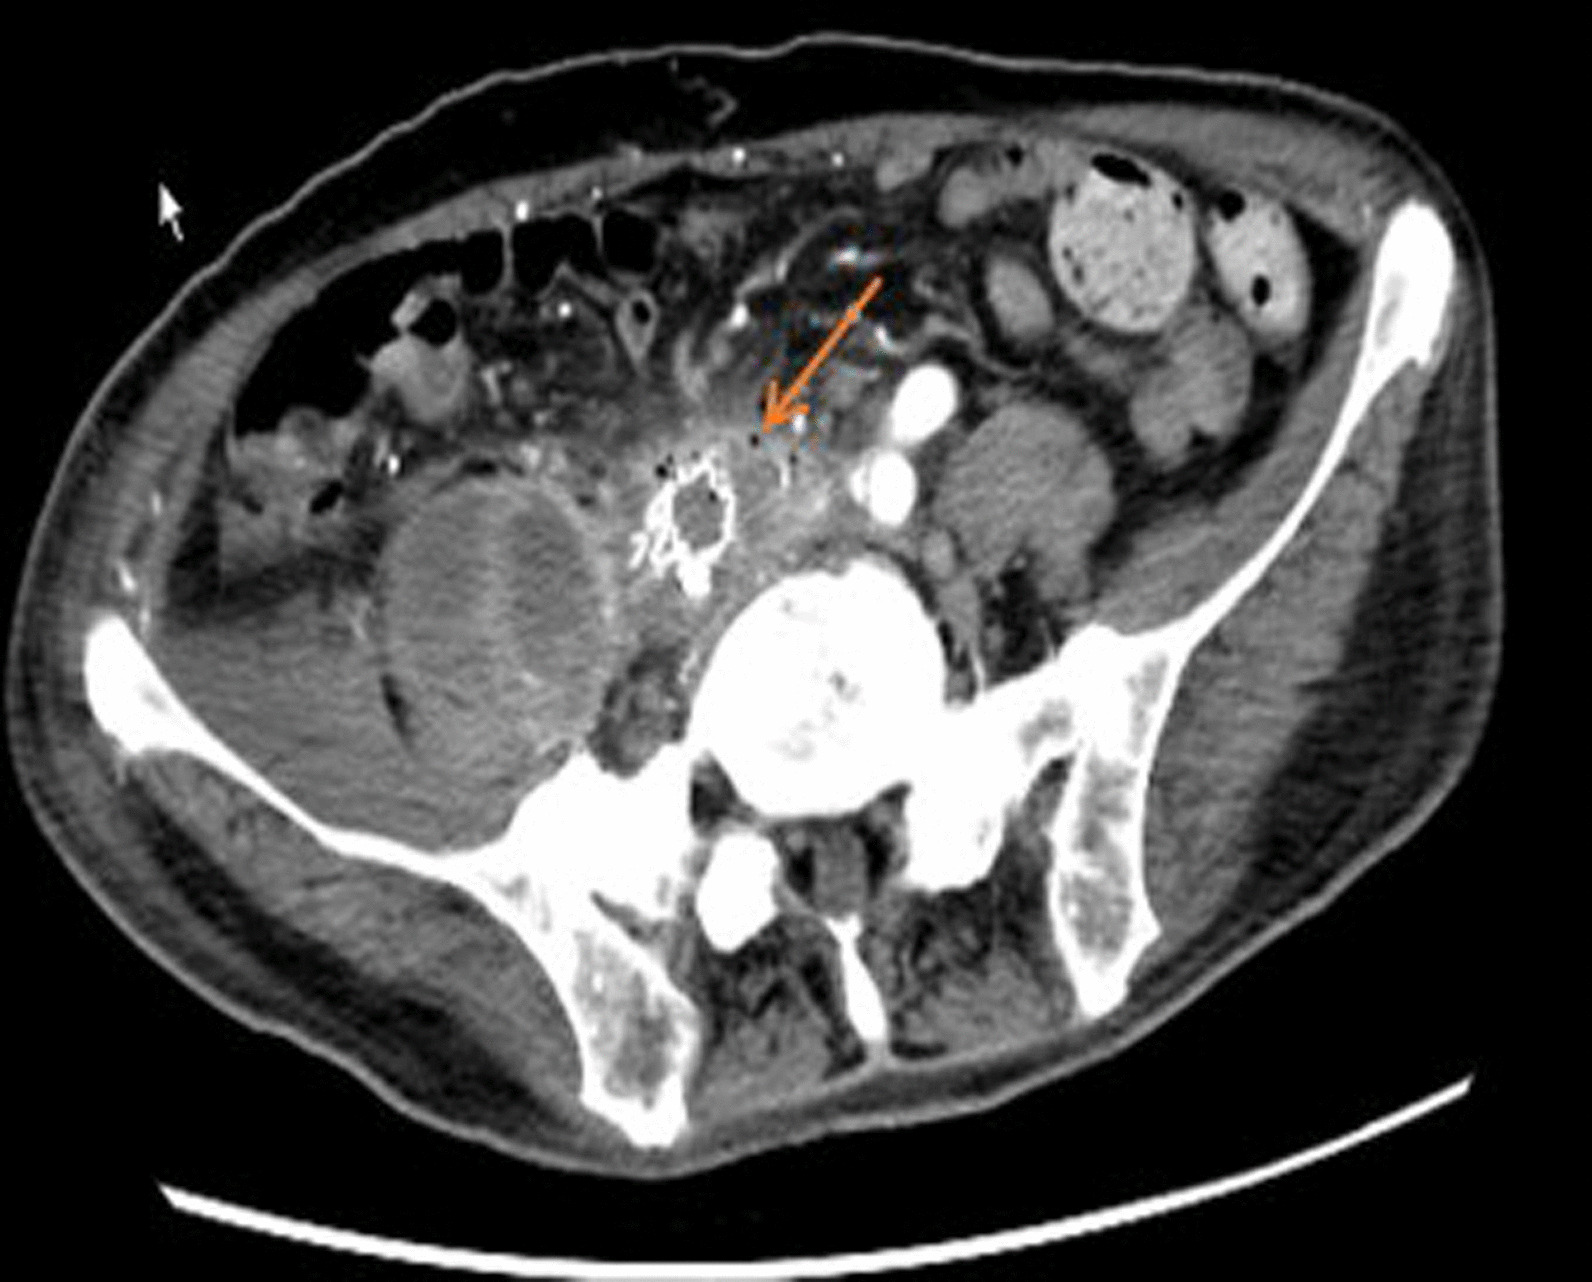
Computed tomography (CT) showed ectopic gas within or adjacent to the right external iliac artery, and equi- or low-density soft tissue collection around the right external iliac artery as well as encapsulated fluid around the right psoas major and iliacus muscles
